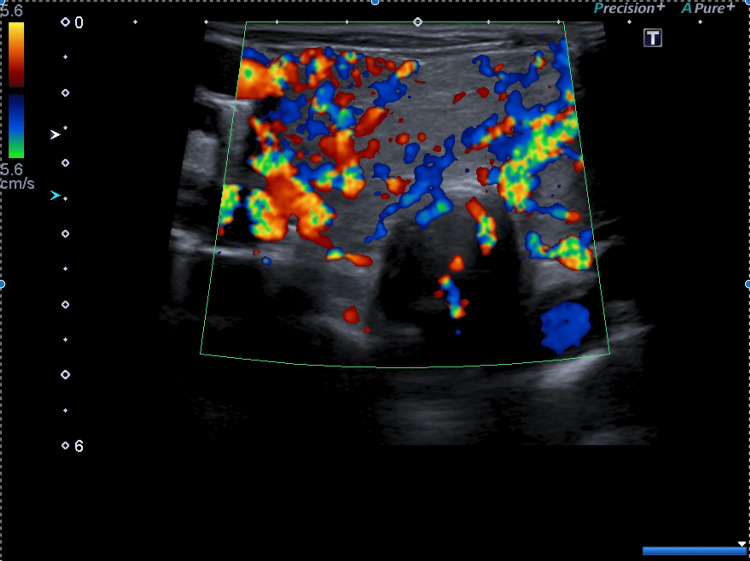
Ecography with Doppler study showing bilateral hypervascularization
